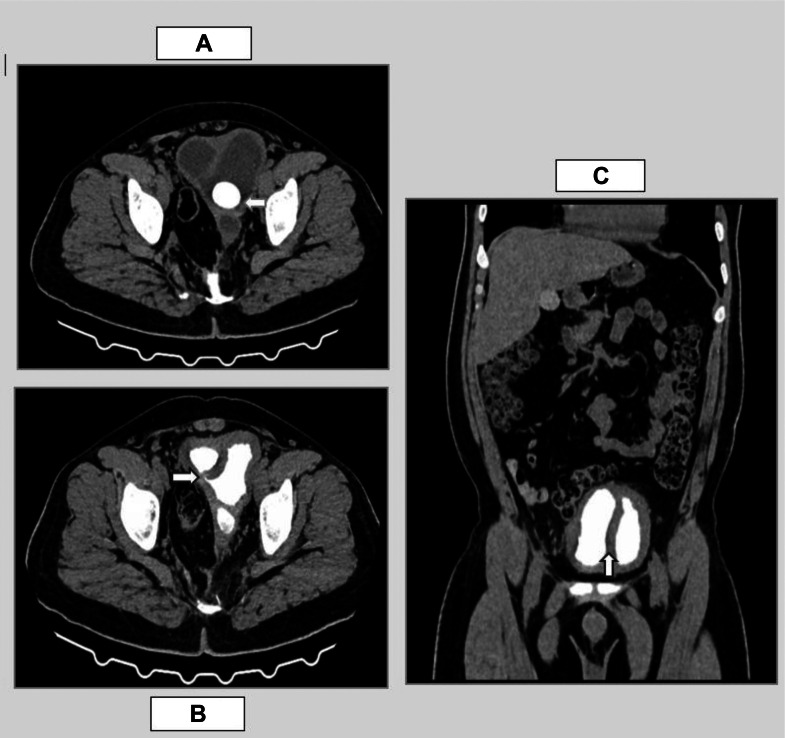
(A) Axial section of plain CT showing a calculus in left compartment of bladder (depicted by white arrow). (B) Axial section of CT urogram showing a small communication between the 2 bladder cavities at the neck of bladder (annotated by white arrow). (C) Coronal section of CT Urogram revealing a thick fibromuscular sagittal septum with attenuation similar to that of bladder wall (Annotated by white arrow) dividing the contrast filled urinary bladder into 2 separate compartments.
